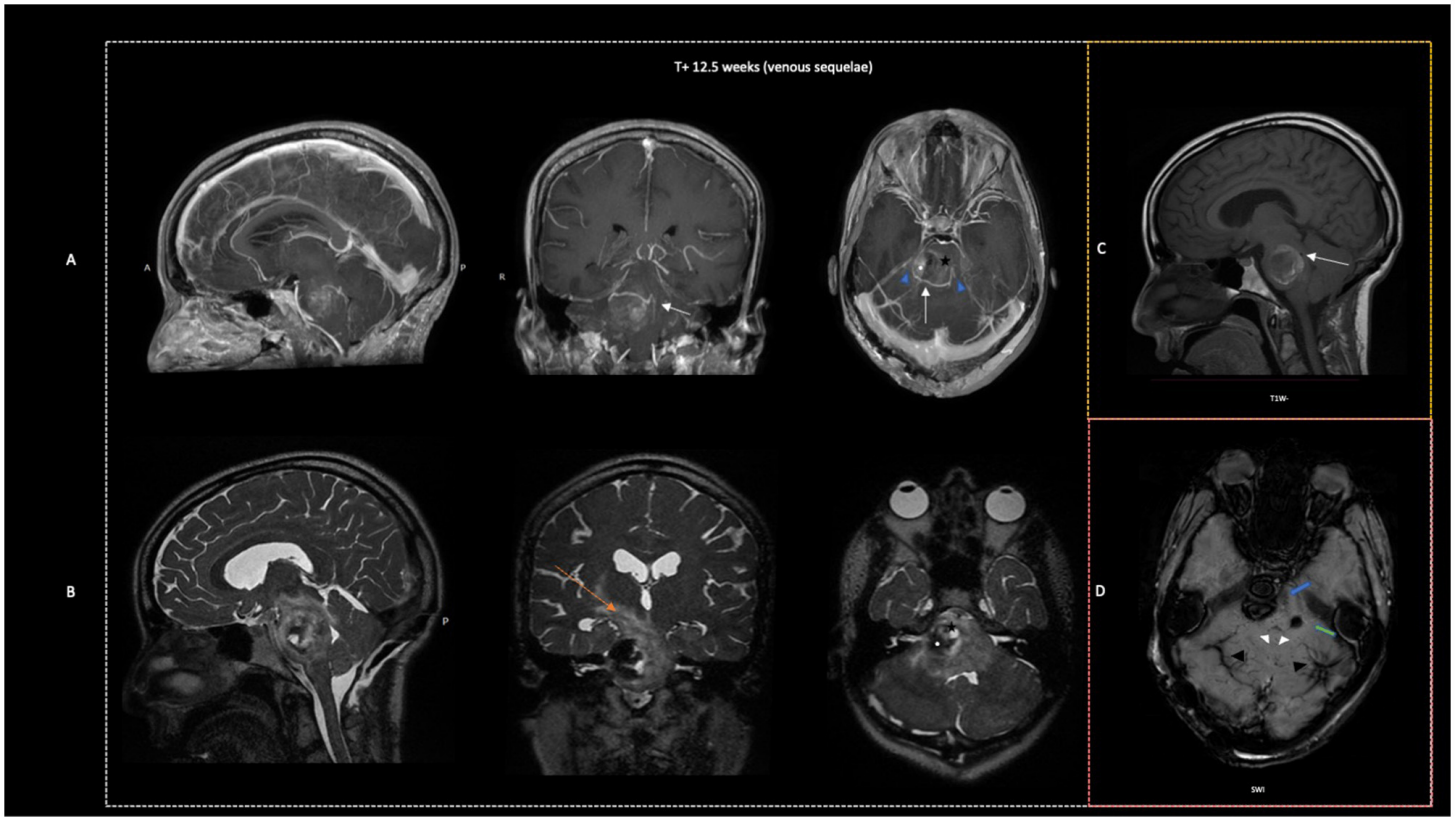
Delayed venous congestion and hemorrhage after the second surgery. Sagittal, coronal, and axial MRI: (A) T1W + Gad; (B) T2W; axial only: (C) TW1 and (D) SWI. Marked edema extending from the pons, middle cerebellar peduncles to the cerebral peduncles and subthalamus (orange arrow) on T2W. Reduction in size of the surgical cavity with a small volume acute hematoma (black star) seen as T1W hyperintense and T2W hypointense signal, and early subacute hematoma (white star) with T1W+ isointense and T2W hyperintense signal. The target CCM (blue arrow) appears to have been resected with no blooming artifact at this location seen on SWI. The contralateral CCM remains (green arrow) on SWI. Reduced filling caliber of main DVA draining vein on T1W+ (white arrow) with outline present but no hypointense signal on SWI. Difficult to comment on the pressure of the DVA system in the presence of marked edema.
